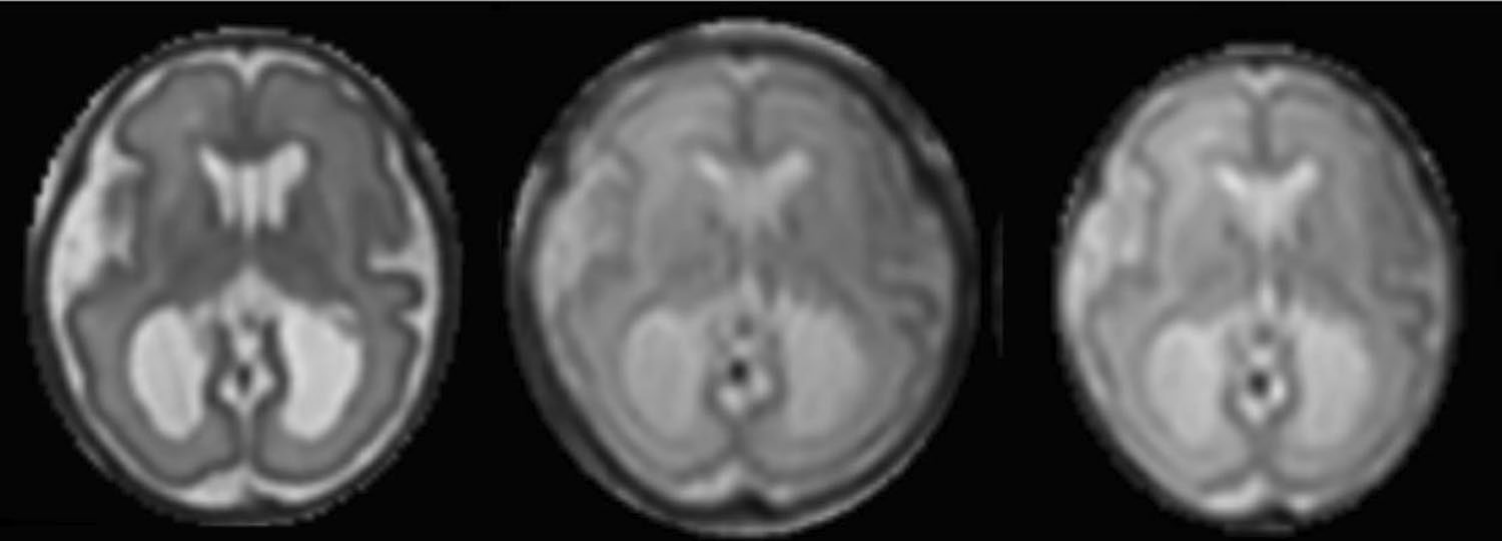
Distortion correction. The reconstructed results are shown for: anatomical T2-weighted (left); *b*_0_ without distortion correction (middle); *b*_0_ after distortion correction (right) (phase-encoding direction: anterior–posterior)
